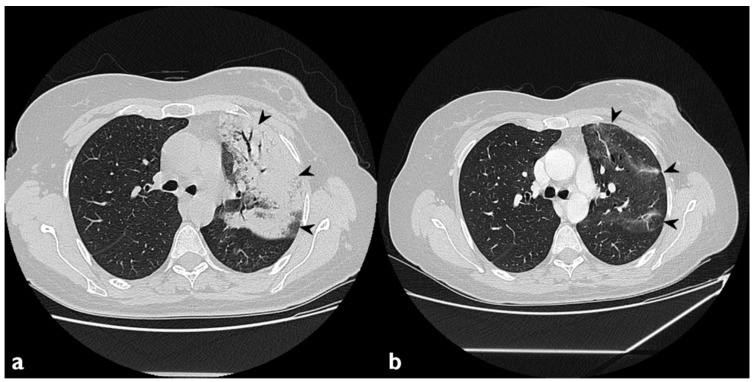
On the left (a): baseline HRCT; left parenchymal consolidations with air bronchogram sign in the context (arrowheads). On the right (b): HRCT after steroid treatment; new ground-glass areas (arrowheads) have appeared in the site of consolidations.
